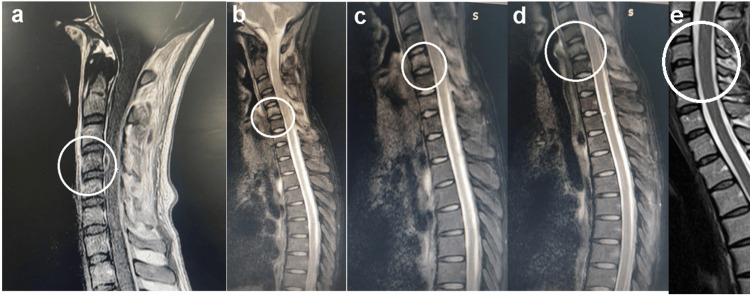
Cervical magnetic resonance imaging of the patient (a) T1 (recognizable by the dark cerebrospinal fluid (CSF)), (b) early T2, (c) T2-sequence showing vertebral enhancement, and (d) showing evidence of vertebral involvement, all revealing lesions at the level of C3, C4, and C5 vertebral levels. (e) shows the healing of the vertebral body after therapy. White circles in (a), (b), (c), and (d) show areas of infection, while those in (e) show healing. Patient identification is removed to ensure privacy.
